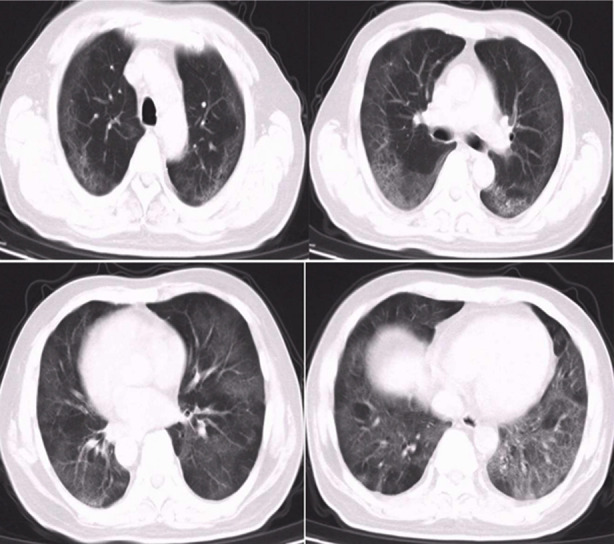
Chest CT scan results after 15 days of hospitalization. Multiple ground-glass lesions were observed, and pulmonary fibrosis was found locally. D-dimer and hs-CRP levels reduced to 0.62 μg/ml and 14.6 mg/L, respectively.
